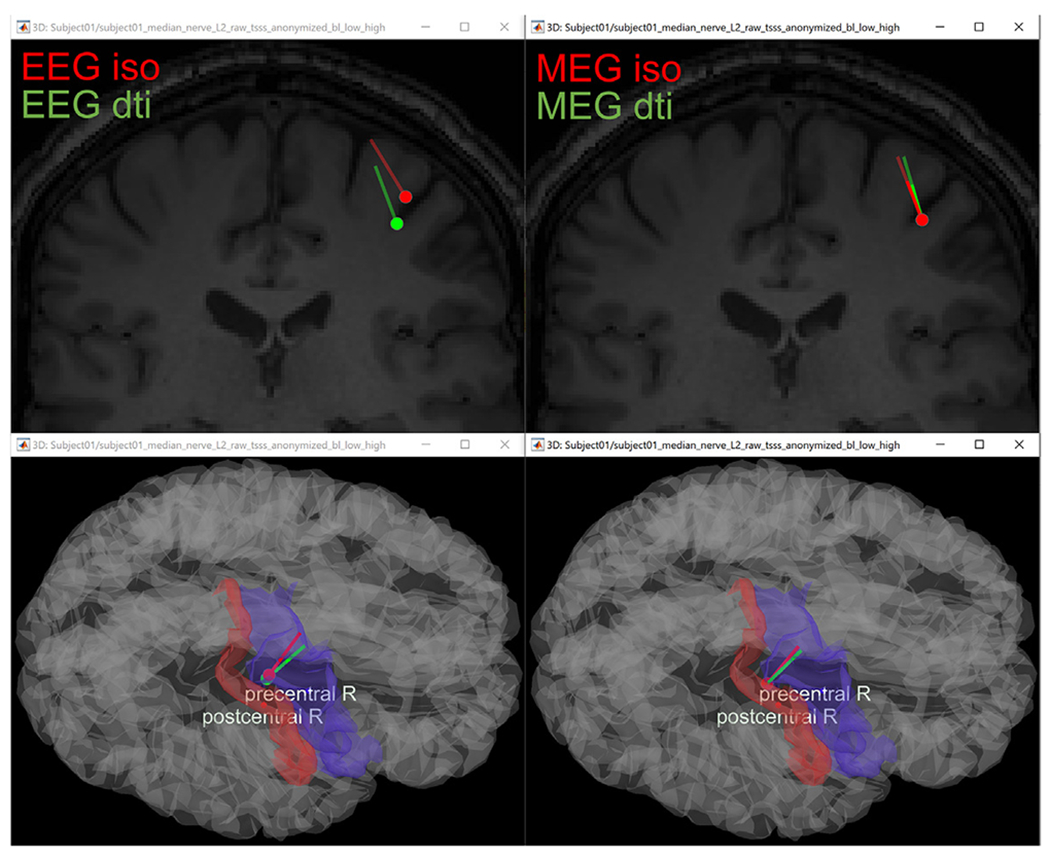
Reconstructed source of the P20 component and its location within the source space displayed on the MRI (top) and cortical surface (bottom). Left: the EEG reconstructed dipoles. Right: the reconstructed dipoles from MEG. Green (red) is the result computed using the head model with anisotropic (isotropic) white matter modeling.
